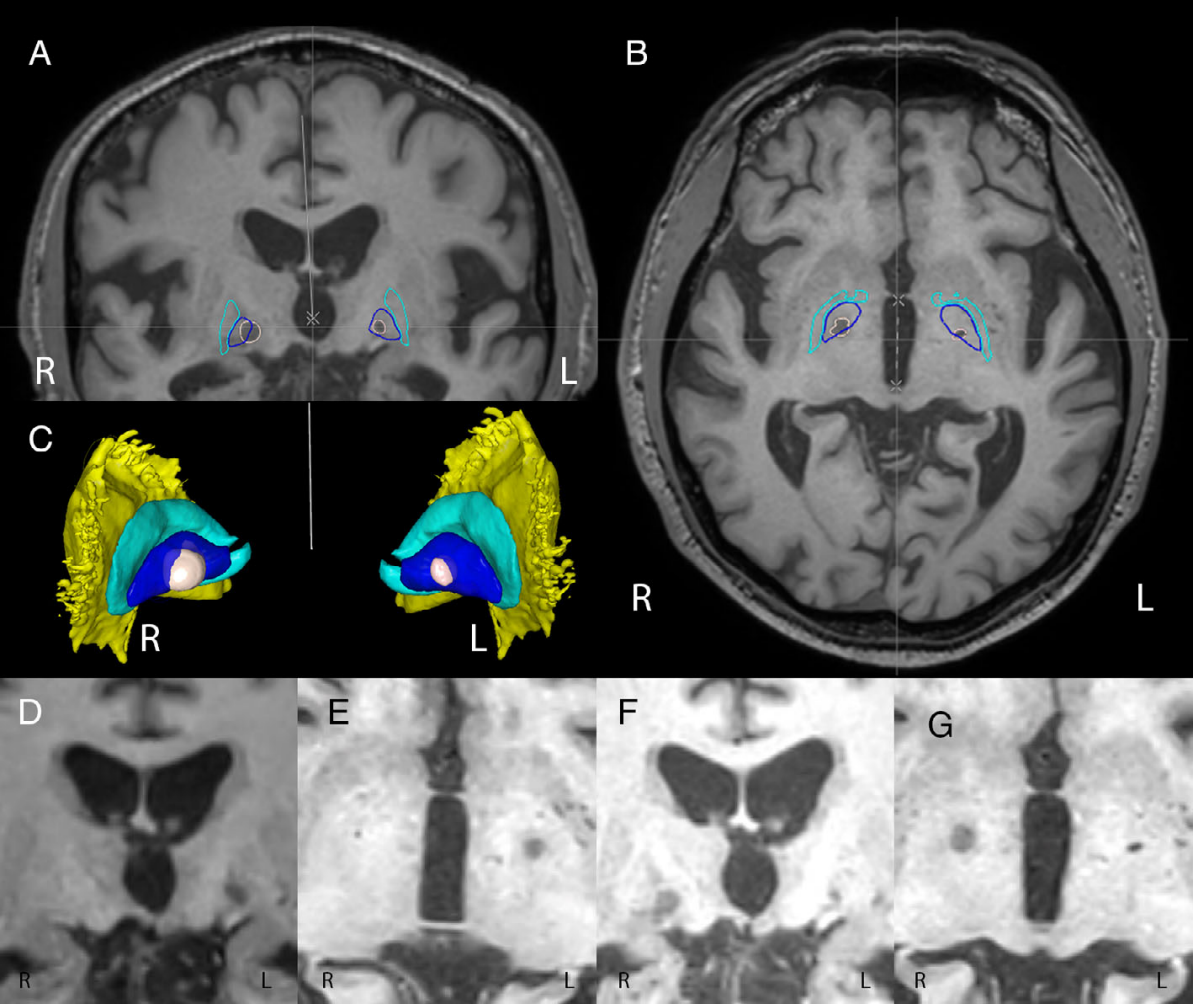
Cranial MRI after bilateral pallidotomies in coronar (A), axial (B) and 3D (C). The ablations are outlined in beige. For better orientation, a 3D‐atlas was superposed using Medtronic SureTune 3.0 (Medtronic, Minneapolis, MN). The GPi is outlined in dark blue, Globus pallidus externus (GPe) in light blue and putamen in yellow (only 3D image). The images (D) and (E) show the fresh left‐sided ablation in the first day postoperatively; the images (F) and (G) show the fresh right‐sided ablation on the first day postoperatively. In all images, letters R and L indicate laterality.
